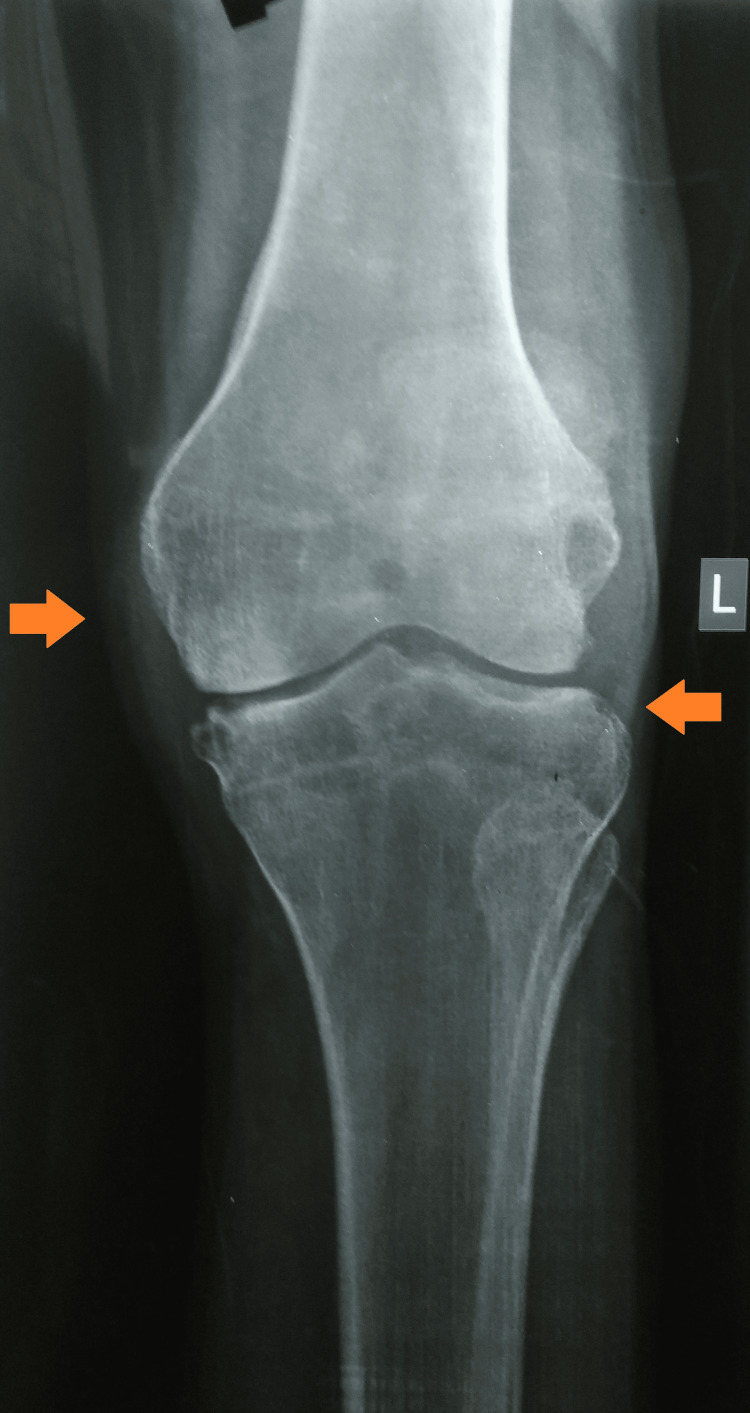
A plain radiograph of the left knee (AP view) was suggestive of knee joint involvement AP: Anteroposterior
